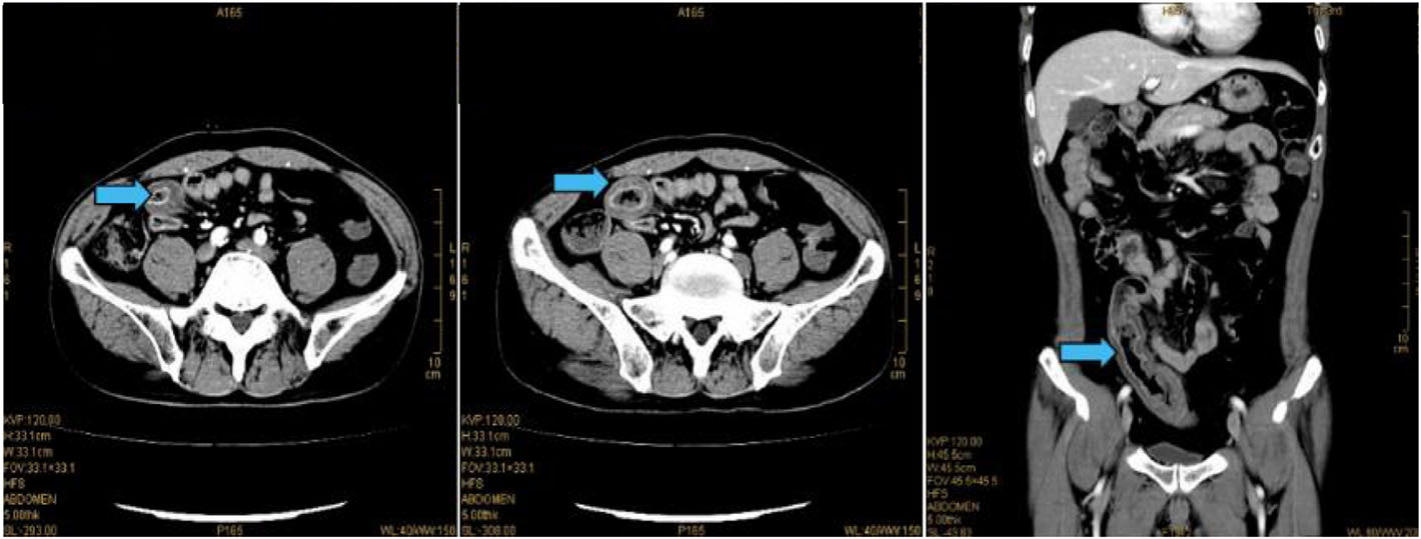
CT scan of the abdomen revealed pelvic ascites with segmental intestinal wall thickening at terminal ileum
